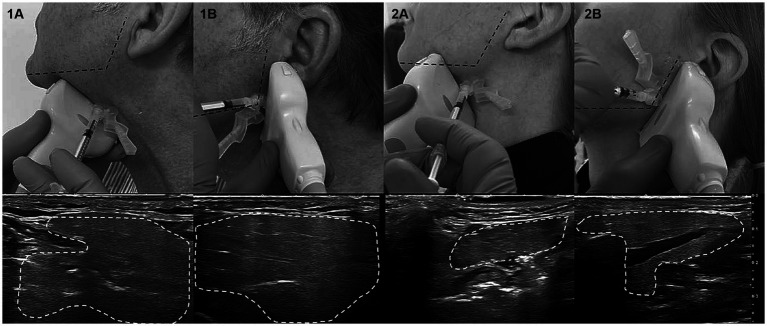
Demonstration of ultrasound-guided injection in subject 1 and 2 on the left side, respectively. (A) Submandibular gland, (B) Parotid gland. (Copyright Tobias Bäumer, Institute of Systemic Motor Science, CBBM, University of Lübeck).
